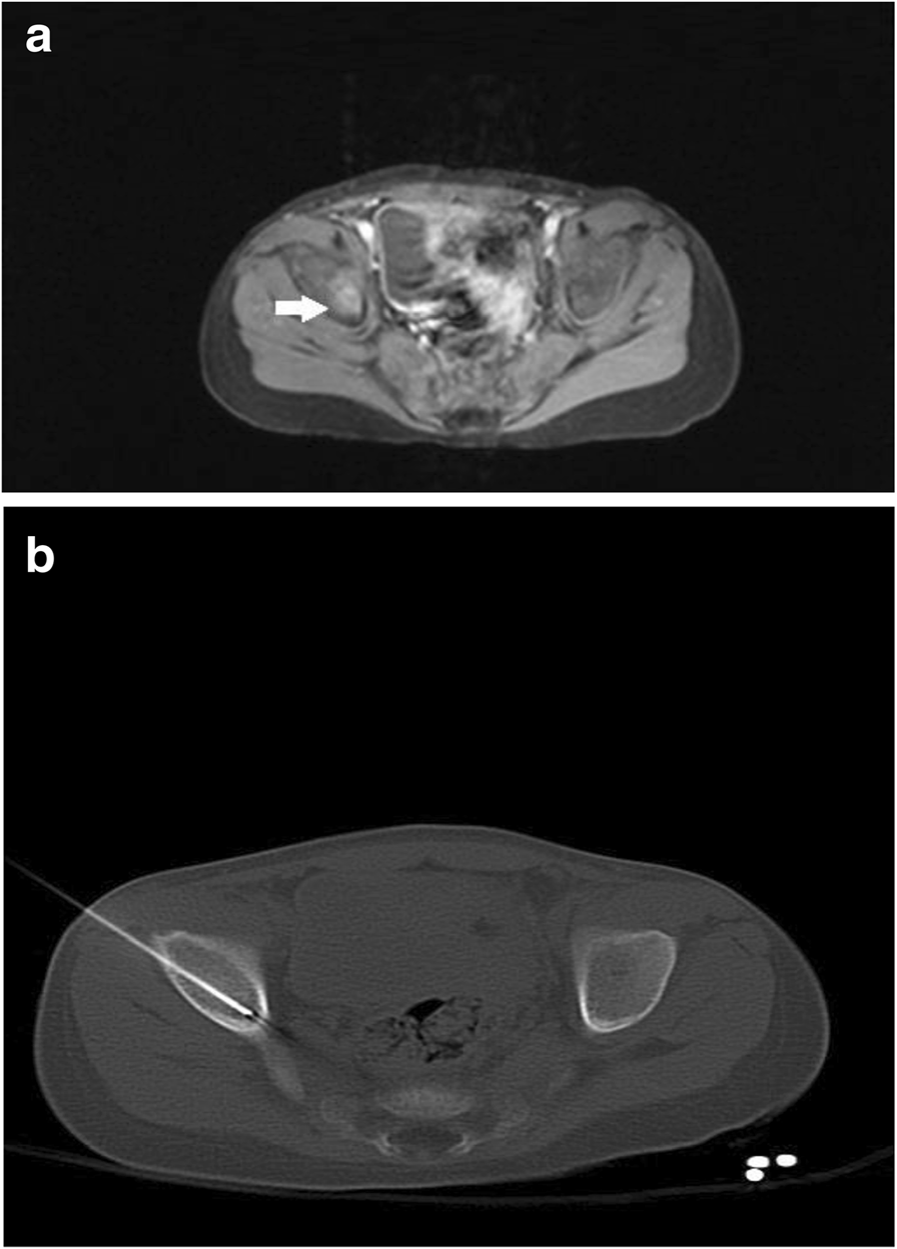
Five-year-old child with neurooblastoma. In April 2012 the patient had in follow up MRI (a) a suspect lesion with a size of 11 × 14 mm in os ileum right. In MRI no further metastatic lesions were documented. The intervention of os ileum was performed with CT guidance. The CT-guided intervention was performed without complications. The pathological report revealed a metastasis from neuroblastoma. a. Enhanced transverse T1-weighted MR image with fat saturation shows a 11 × 14 mm suspect lesion in os ileum right (*arrows*). b. CT-Image documented a location of biopsy needle in the lesion of os ileum
